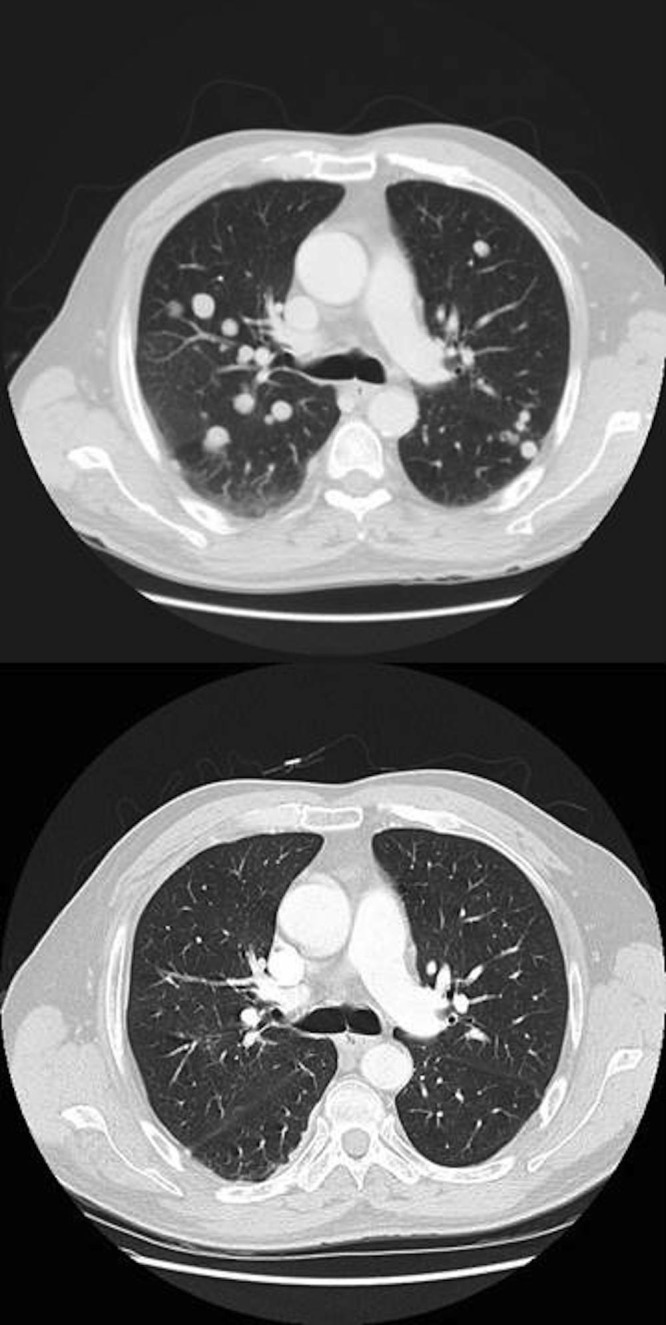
The axial CT scans of the thorax before and after radiation of the liver. Figure 1A (top) The February 2010 axial CT thorax demonstrates multiple metastases before radiation . Figure 1B (bottom) The August 2010 axial CT thorax demonstrates resolution of metastases five months after radiation .
